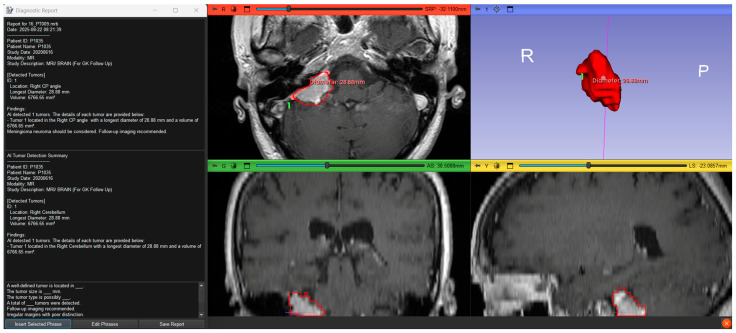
Representative AI assistance interface in 3D Slicer. The left side shows structured lesion-level information used during physician report preparation, and the right side shows MRI-based lesion localization and contouring support.
